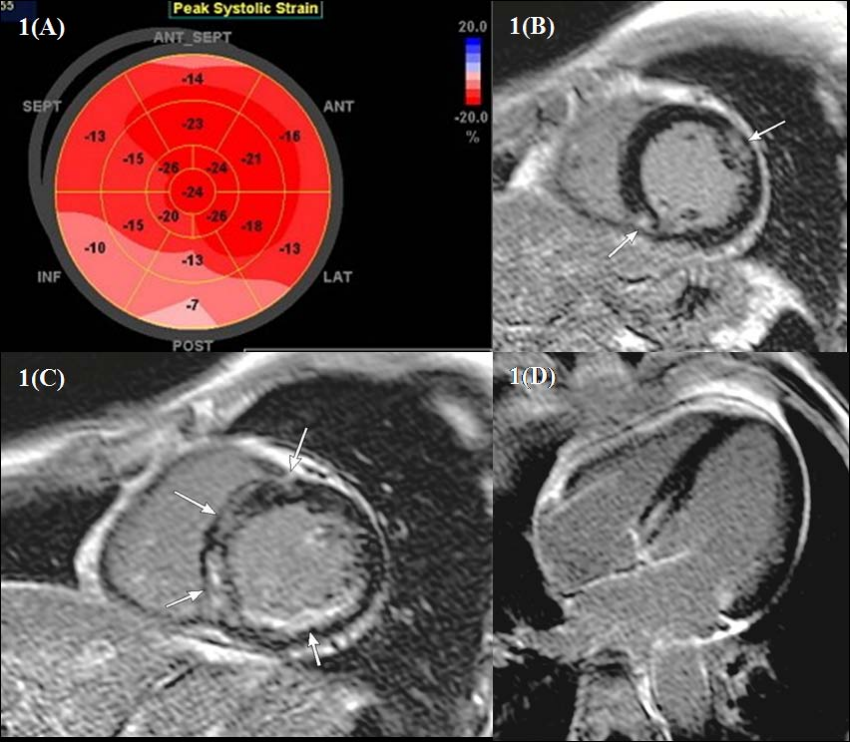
Patient #4: (A) Automated function imaging (AFI) segmental PSLS by Echocardiography. Segmental PSLS was decreased in inferior and basal anteroseptal area. (B-D) Cardiovascular Magnetic Resonance. (B,C) Late gadolinium enhancement images in the short-axis view (delay 10 minutes). There was fibrosis in inferior and basal anteroseptal area (arrow). (C) Late gadolinium enhancement images in the four-chamber view (delay 20 minutes). There was fibrosis in inferoseptal area (arrow).
